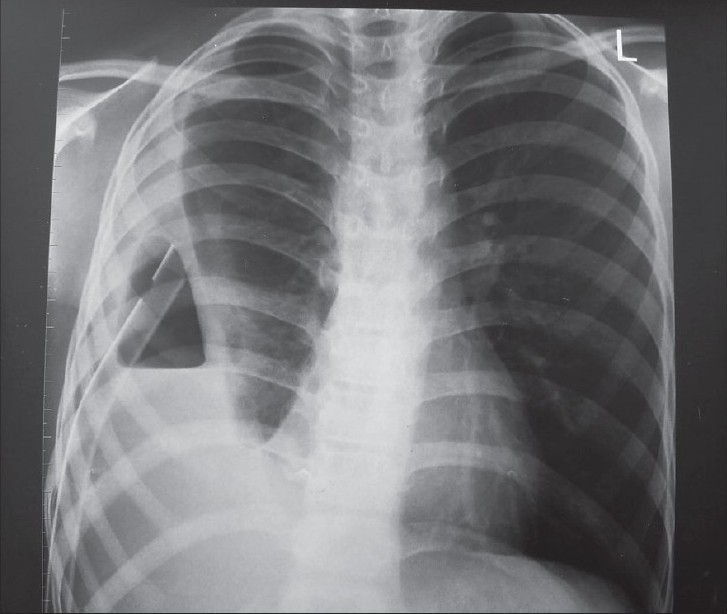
Chest X-ray: Thick pleural peel not allowing full expansion of the right lungPleural debris>20% volume<20% volume but symptoms (fever/tachypnea) are persistentMultiloculated empyema [Figure 9]
